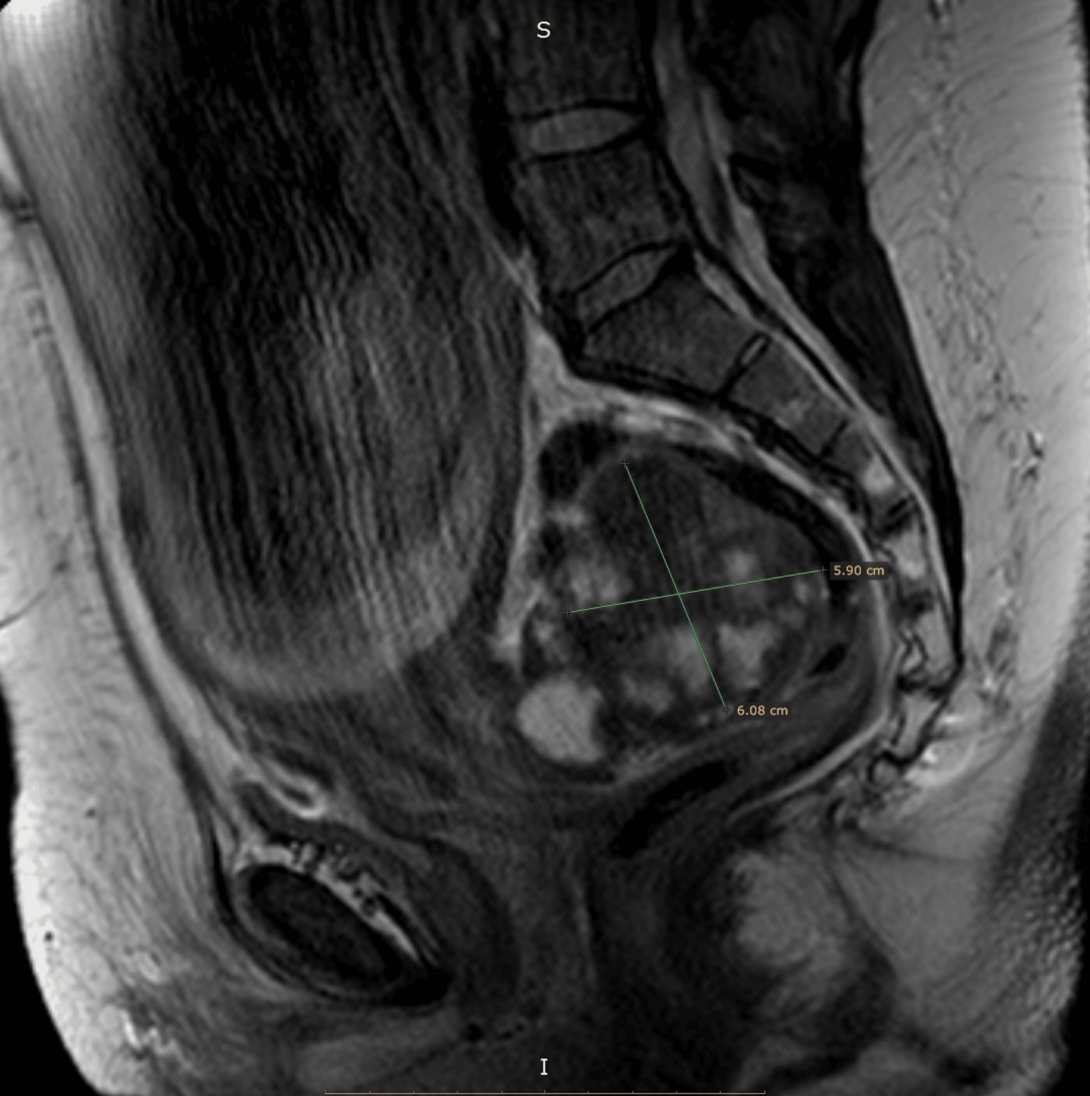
MRI at 28 weeks of gestation, dimensions of the tumor and location (sagittal plane) MRI, magnetic resonance imaging.
